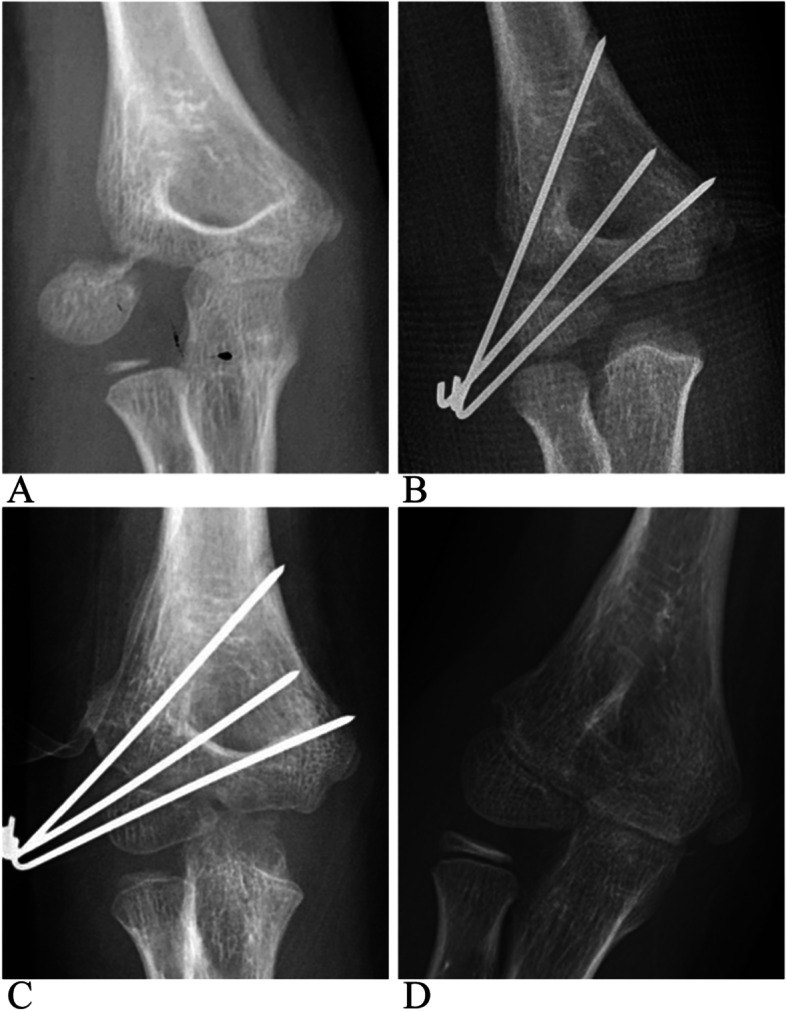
A Song stage 5 and Milch typeIILCHF of an 11 years old boy. A Pre-operative anterior-posterior X-ray. B Post-operative X-ray after CRPP. C X-ray 6 weeks post surgery. D X-ray 11 months post surgery
